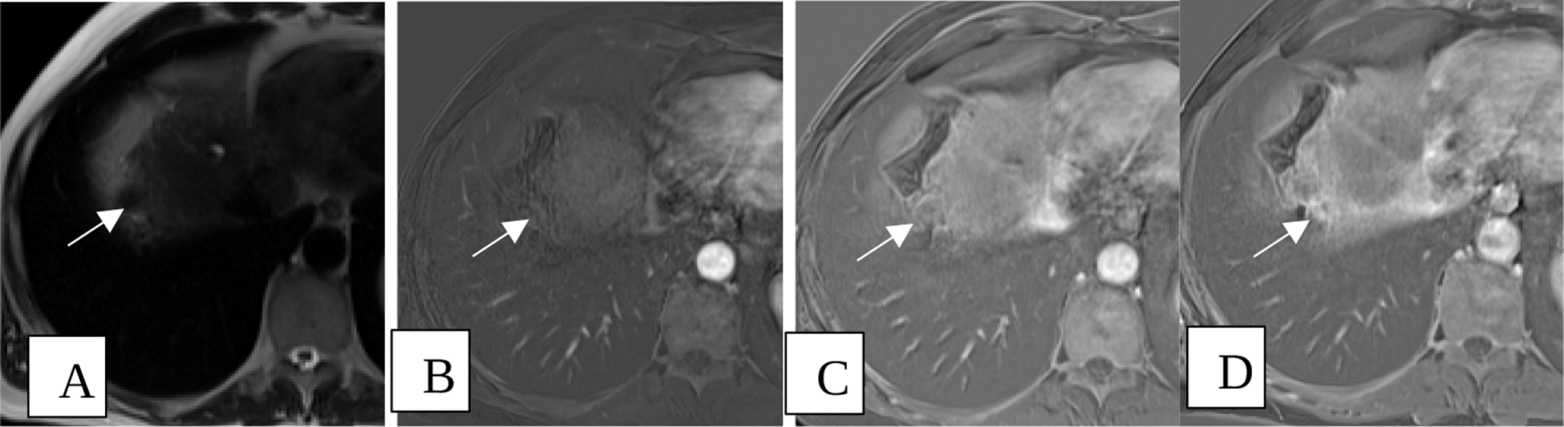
55-year-old male with cirrhosis, status post TACE for a 2.3 cm LI RADS 5 observation in segment VIII. Multiphasic MRI of the abdomen with axial T2 weighted images demonstrating a T2 hypointense lesion at the right hepatic dome (A). T1 weighted images were obtained before and after intravenous gadolinium contrast with subtractions performed in arterial (B), venous (C) and 5 min delayed phases (D) demonstrating increasing internal contrast enhancement within the cavity (arrows) in keeping with LR-TR equivocal
